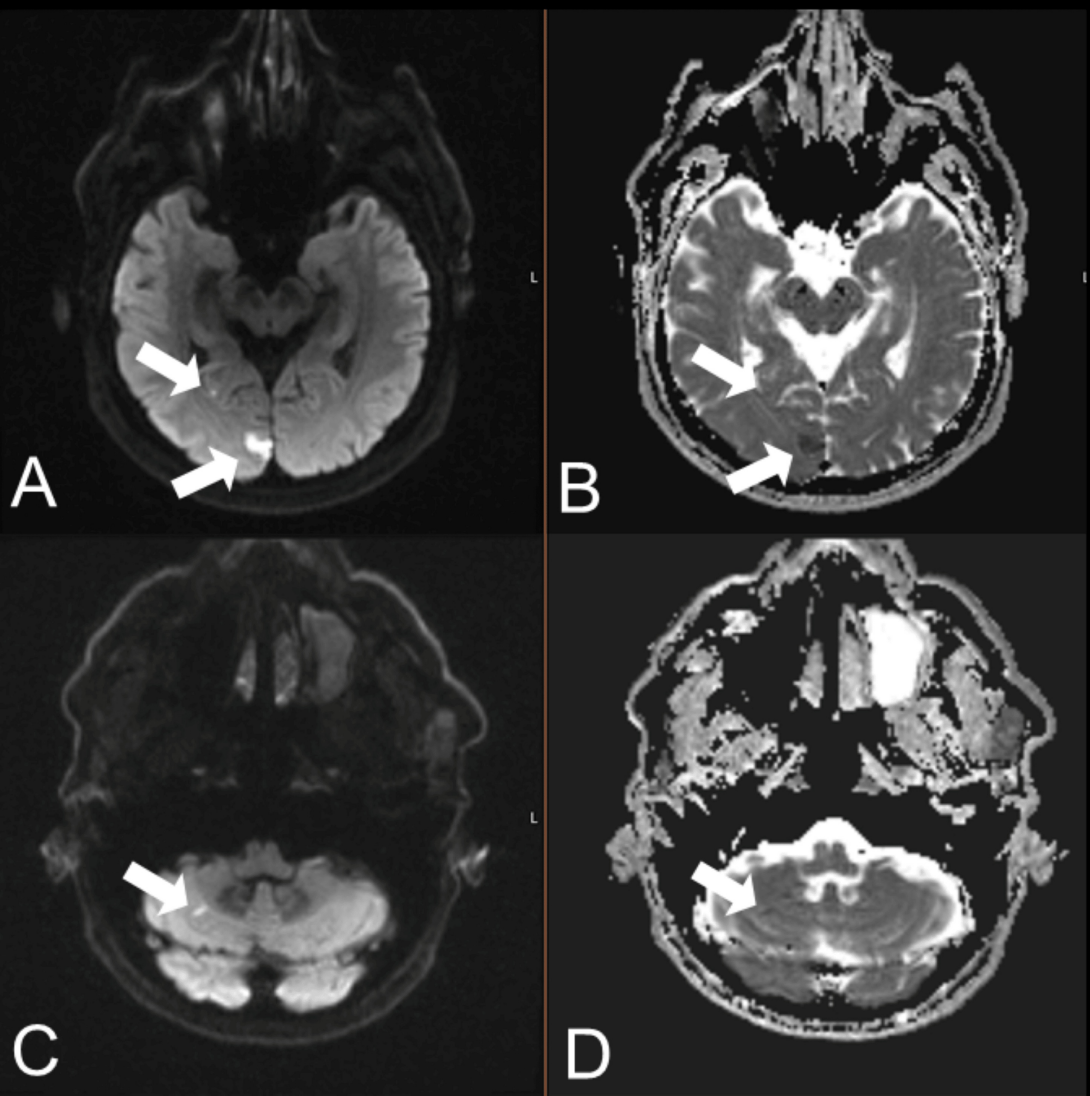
Brain MRI in the axial plane. A and C: DWI sequences; B and D: Corresponding ADC sequences. The images demonstrate multiple small acute ischemic infarcts showing restricted diffusion (hyperintensity on DWI and corresponding low signal on ADC) in the right occipital lobe, right posterior temporal lobe, and right cerebellum (arrows). These infarcts are within the vascular territories of the right posterior cerebral artery and the right superior cerebellar artery. DWI: diffusion-weighted imaging, ADC: apparent diffusion coefficient
